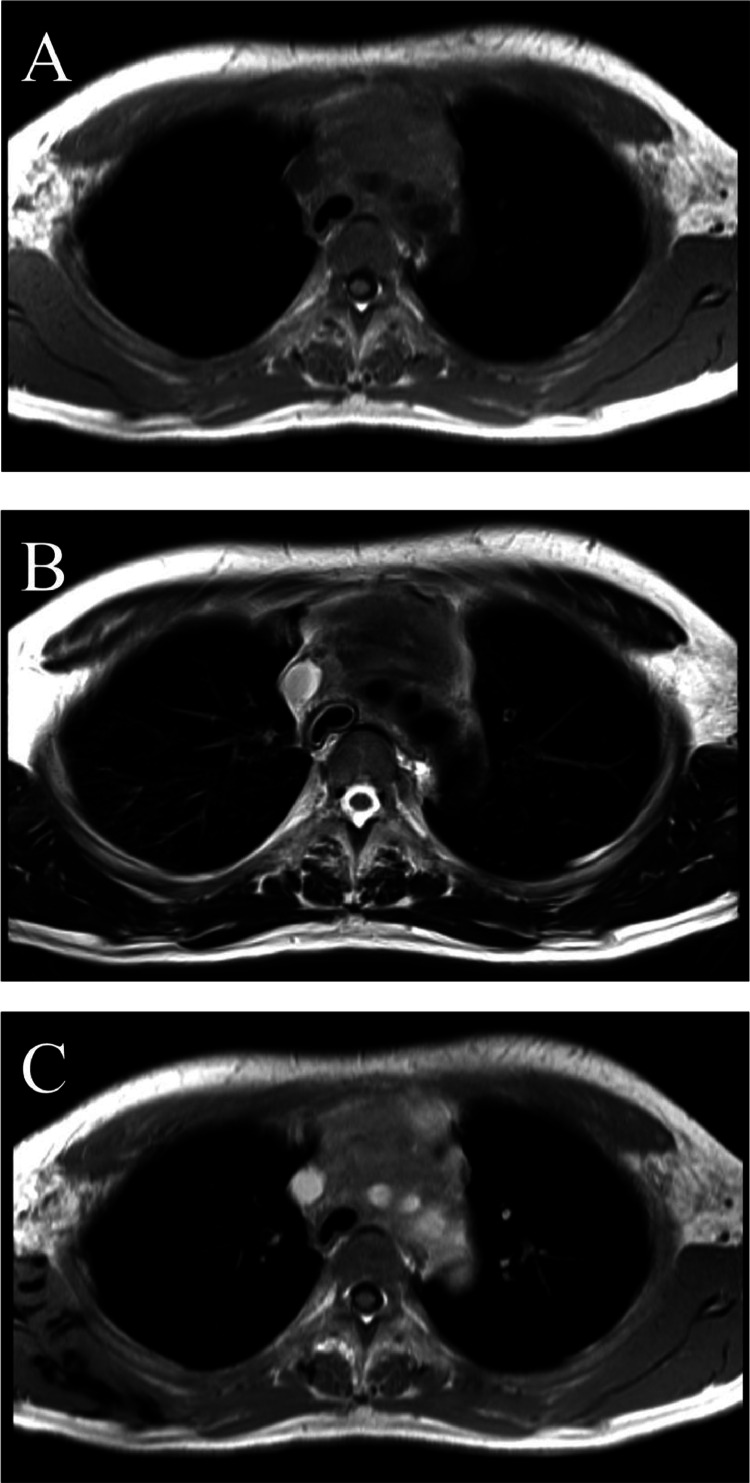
Axial MRI of T1WI(A), T2WI(B), T1WI after injection of Gadolinium (C) in the second case. Axial MRI shows a mediastinum mass with an iso signal on T1-weighted images(A) and a slightly high signal on T2-weighted images(B). Contrast-enhanced MRI showing homogeneous enhancement(C).
